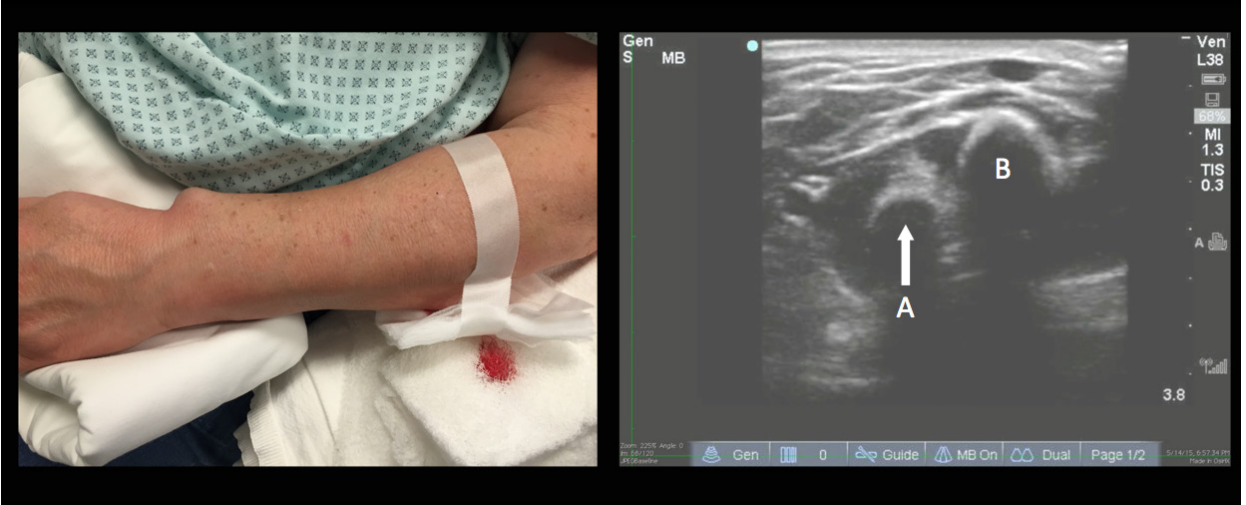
Open left forearm deformity with sagittal ultrasound image of the left forearm demonstrating a foreign body (A) radial to the ulna (B).
